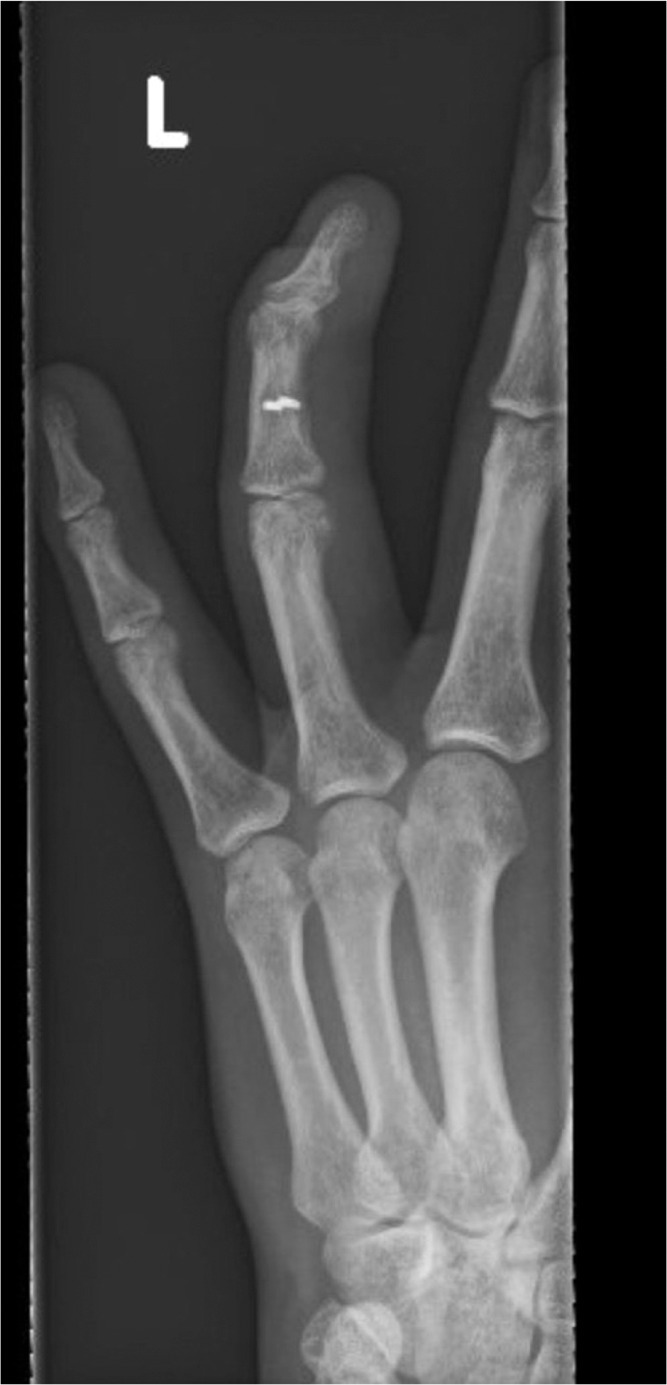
Oblique X-Ray of right hand (Case 2: Post-op).
